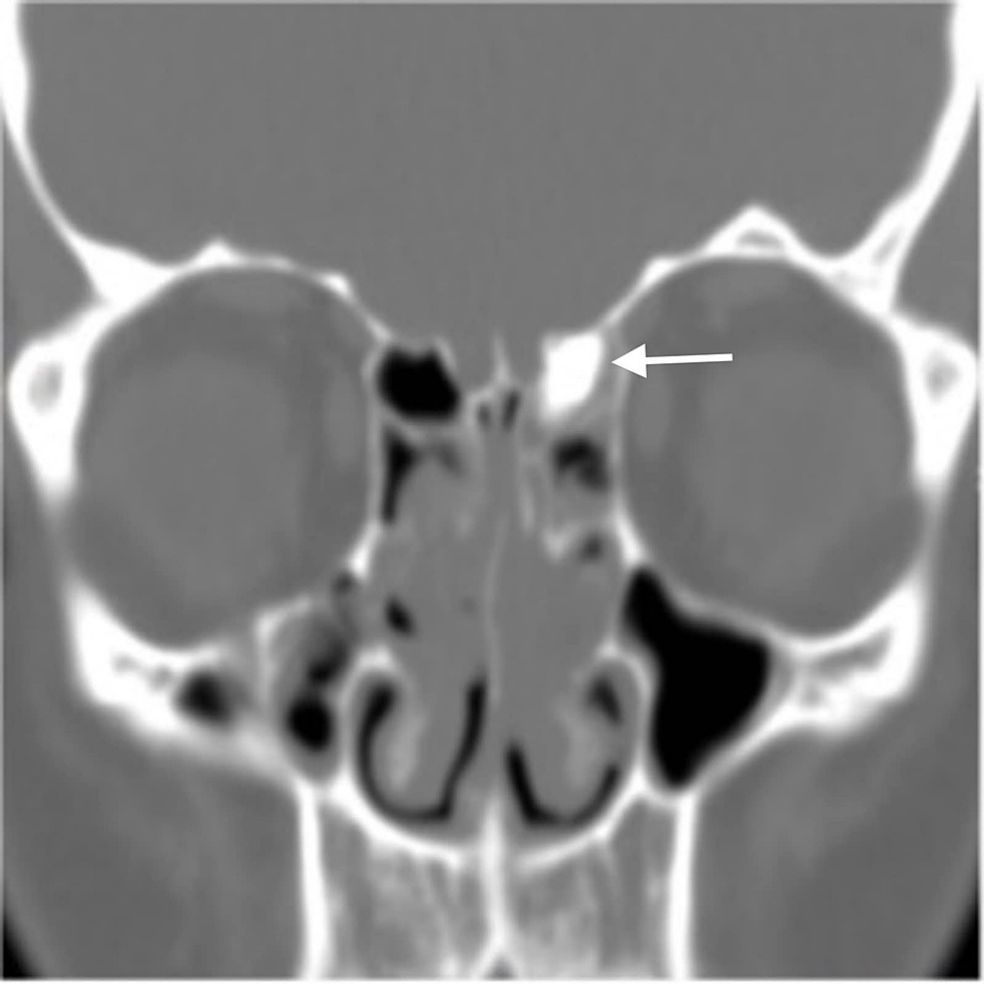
Old CT scan of the patient (performed in 2008): coronal view shows small hyperdense lesion within left ethmoid sinus.
